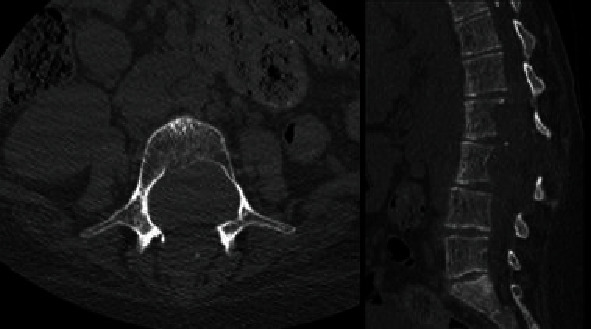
A lumbar spine CT scan: axial and sagittal views.
